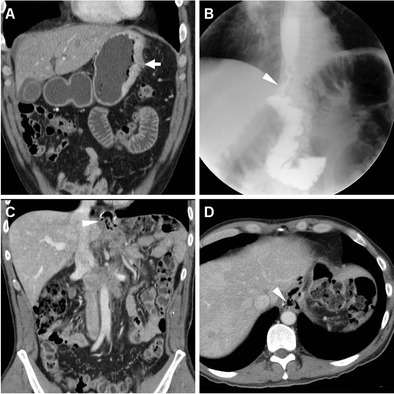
In a 39-year-old male with endoscopic and bioptic diagnosis of stomach adenocarcinoma, preoperative staging CT with oral water distension (A) showed marked solid, enhancing mural thickening from the gastric fundus along the greater curvature (thick arrow). On the fifth postoperative day (POD) after uncomplicated total gastrectomy with lymphadenectomy and splenectomy, contrast fluoroscopy (CF, B) showed normally patent, thin EJS (arrowhead) with opacification of jejunal limb and no anastomotic contrast medium leakage. Corresponding expected postsurgical contrast-enhanced CT (C, D) appearances at hospital discharge included stapled EJS (arrowheads) without peri-anastomotic air or fluid, absent spleen without collections in the surgical site
